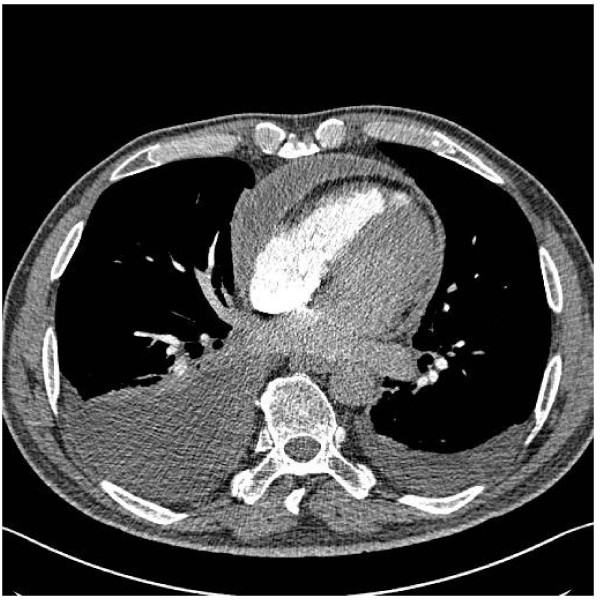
CT Chest showing thickened pericardium.
